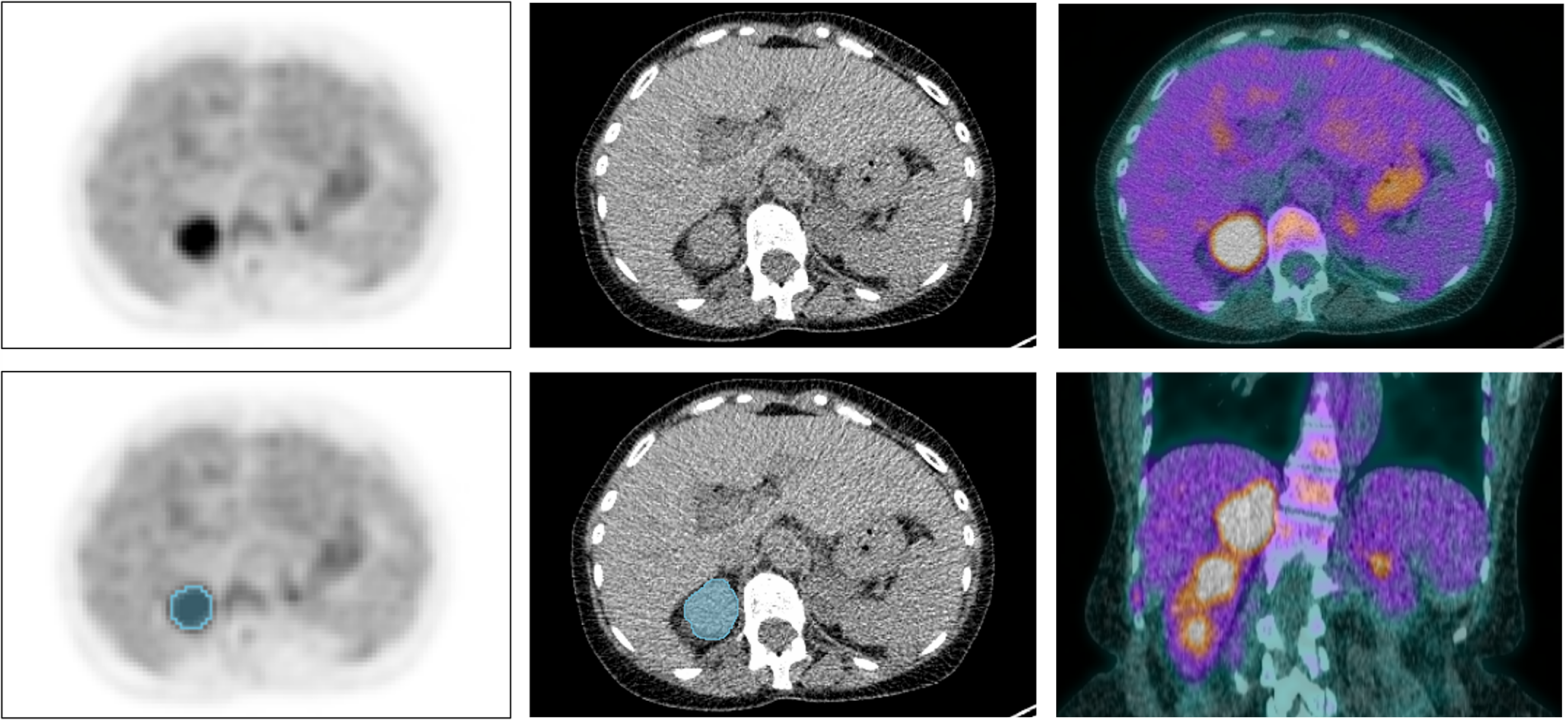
[^18^F]FDG-PET/CT scan of patient with a sporadic pheochromocytoma in the right adrenal. The blue area denotes the volume of interest, SUV_max_ = 7.16 g/mL, SUV_mean_ = 4.76 g/mL, metabolic tumour volume = 25.97 cm^3^
